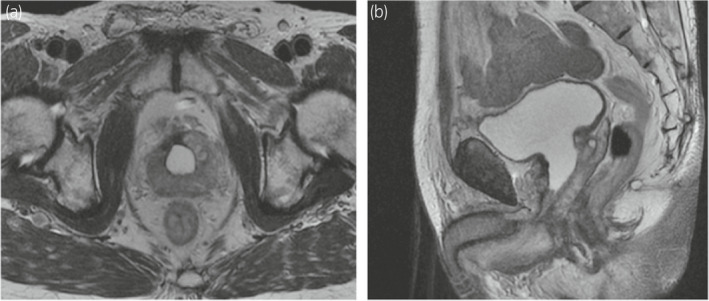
Magnetic resonance imaging prior to the start of brachytherapy––horizontal (a) and sagittal (b) views.
